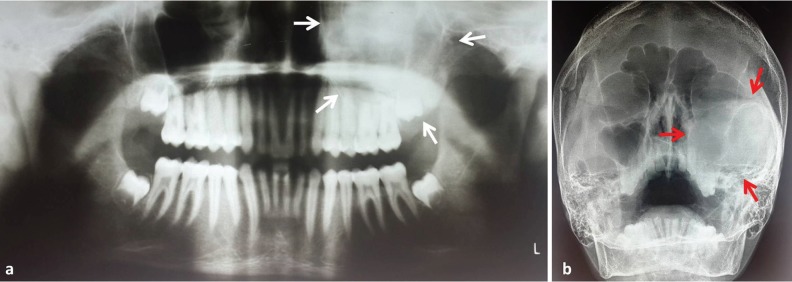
Orthopantomogram (a) and paranasal sinus view (b) showing homogenous radiopaque lesion of left maxilla.
